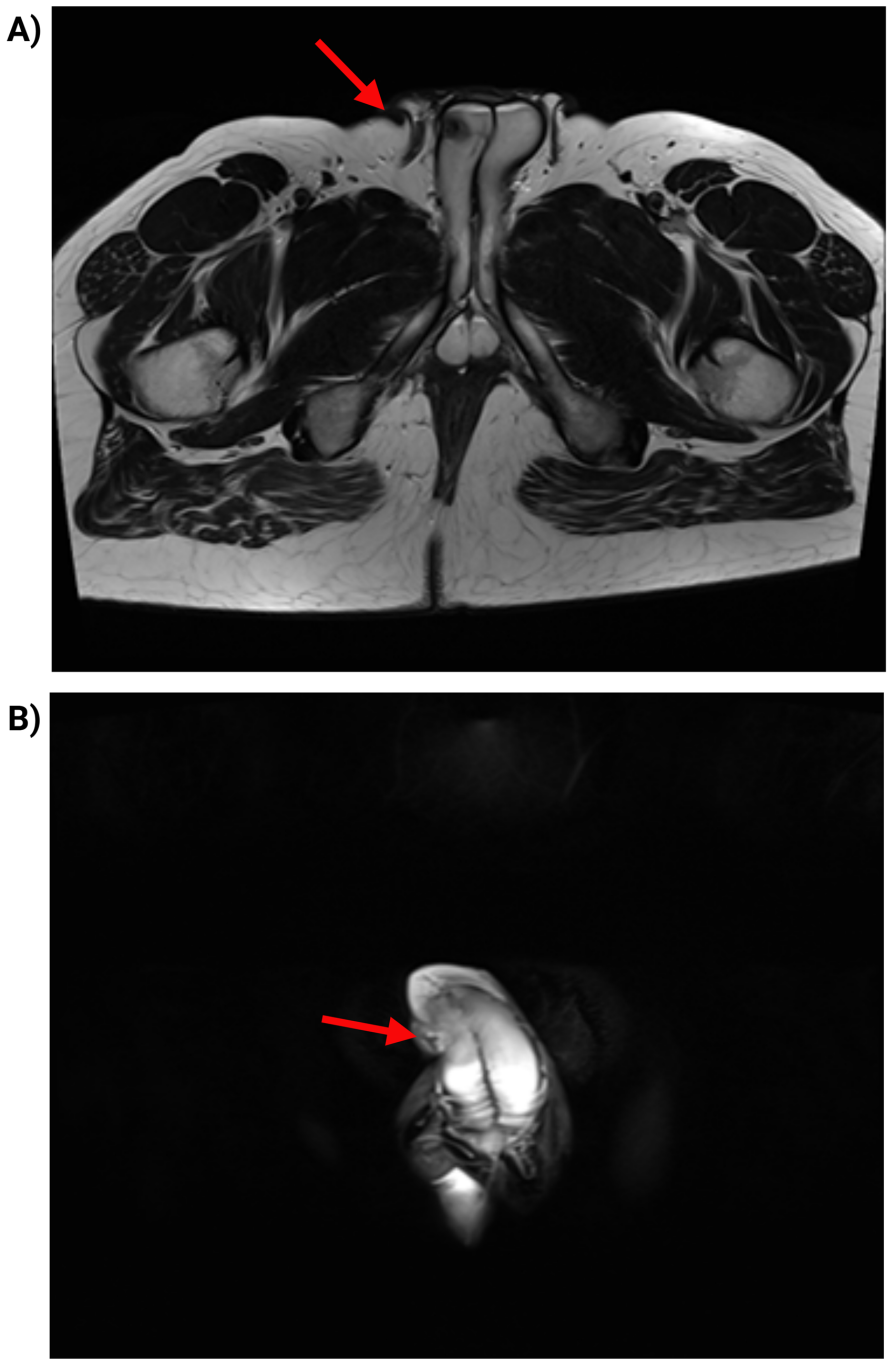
(A) T1-weighted MRI sequence demonstrating well-defined mass extending from the ventral penile shaft and infiltrating the corpus spongiosum, corpora cavernosa, and the glans penis, with a hypointense signal (arrowhead). (B) T2-weighted MRI sequence showing a nodular hyperintense lesion at the level of the penile shaft (arrow).
